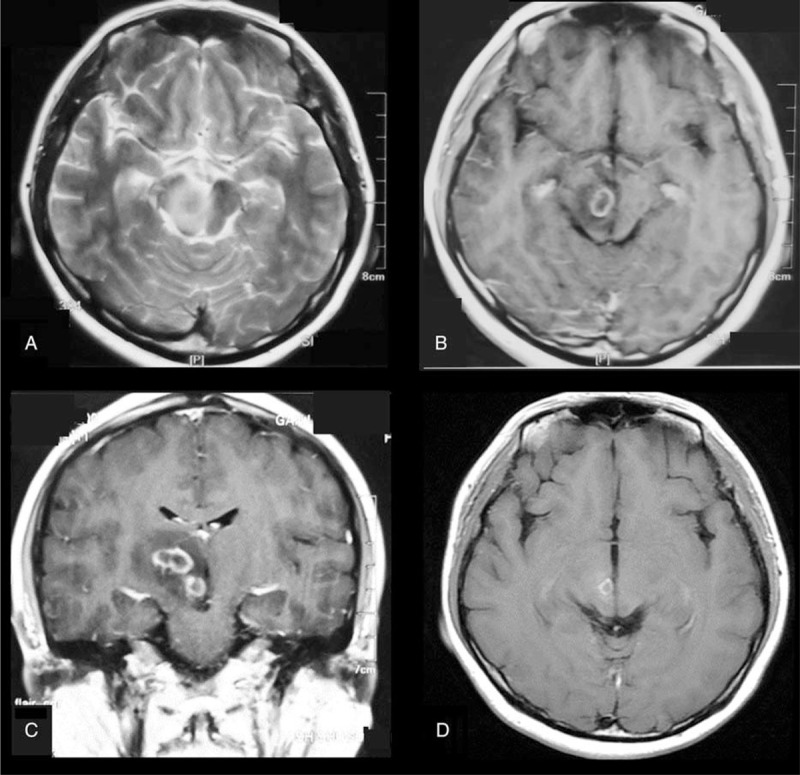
MRI findings in 2016 Axial T2WI (A) and post contrast T1WI (B) showed a rim-enhanced lesion in the right midbrain surrounded by vasogenic edema. Sagittal post contrast T1WI (C) revealed 2 masses in the right midbrain and thalami. Post contrast T1WI 1 month later (D) showed resolution of the lesion.
